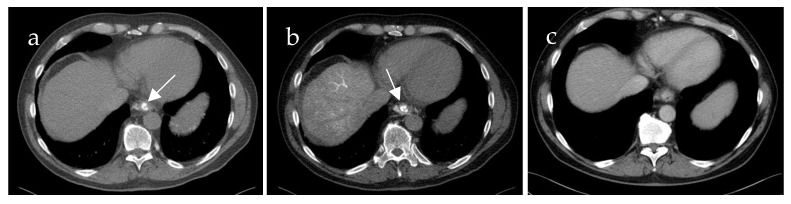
Patient of 61 years of age with portal hypertension and isolated portal vein thrombosis without involvement of the confluence. The computed tomography spleno-portography (a) shows contrasted esophageal varices (EV). The computed tomography mesenterico-portography (b) also shows contrasted EV. The EV are therefore fed by both the superior mesenteric vein and the splenic vein (white arrows). Contrast-enhanced computed tomography (c) after intravenous injection of contrast medium shows low contrast of all tissues and vessels. It does not reveal clearly distinguishable varices in the esophagus.
